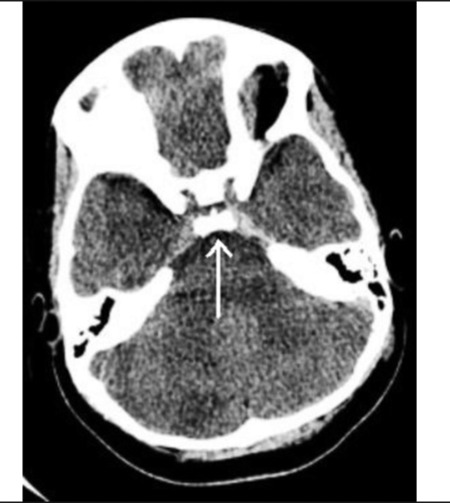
Computed tomography (CT) scan of brain on post-operativeday 1 demonstrating the catheter tip just above the level of the sellaturcica. Arrow points to catheter tip
